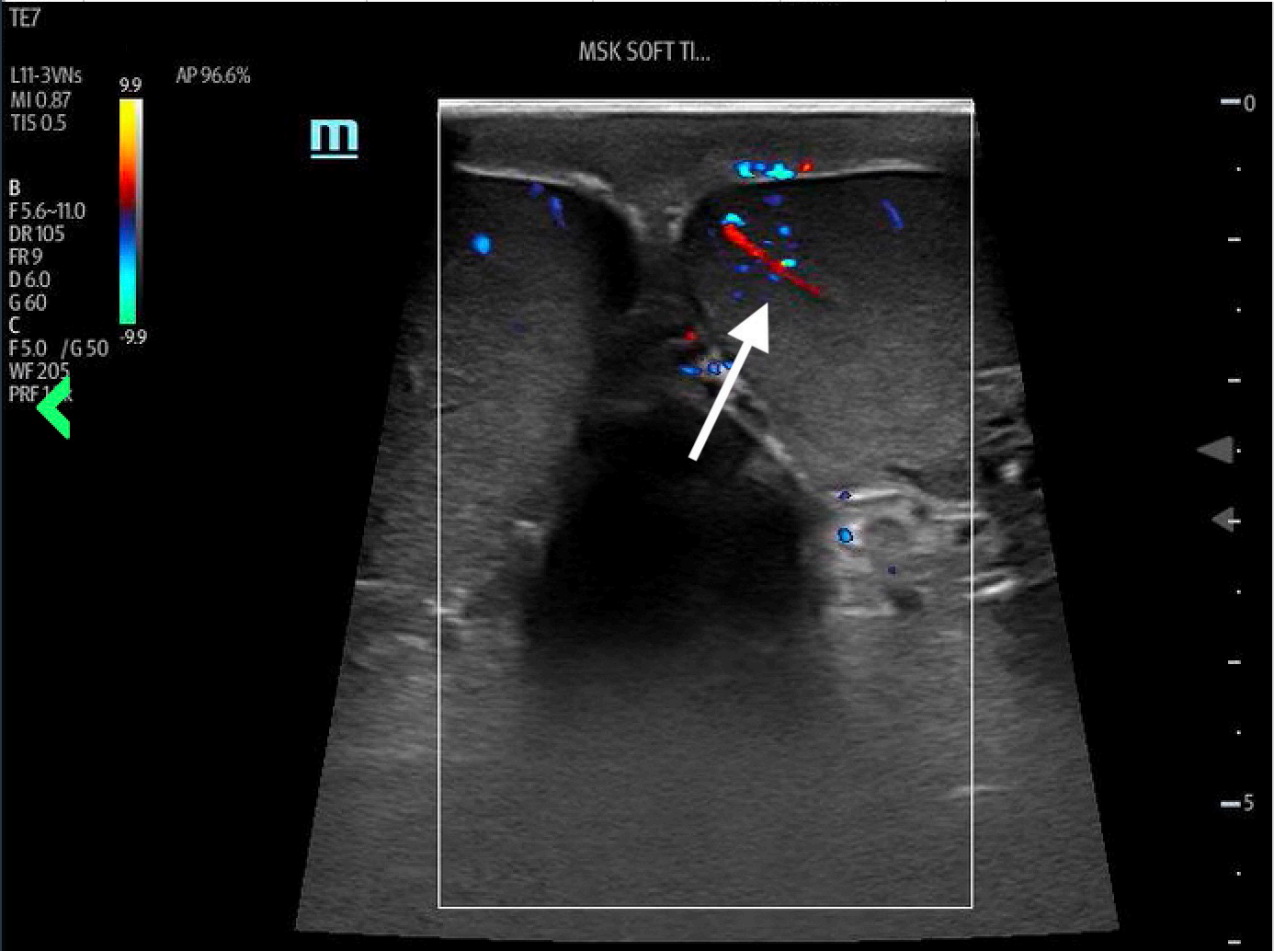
Side-by-side view of testicular assessment with point-of-care ultrasound after manual detorsion. The testicle on the image-right corresponds to the patient’s left testicle, with bilateral appreciable Doppler flow highlighted by the included arrow.
